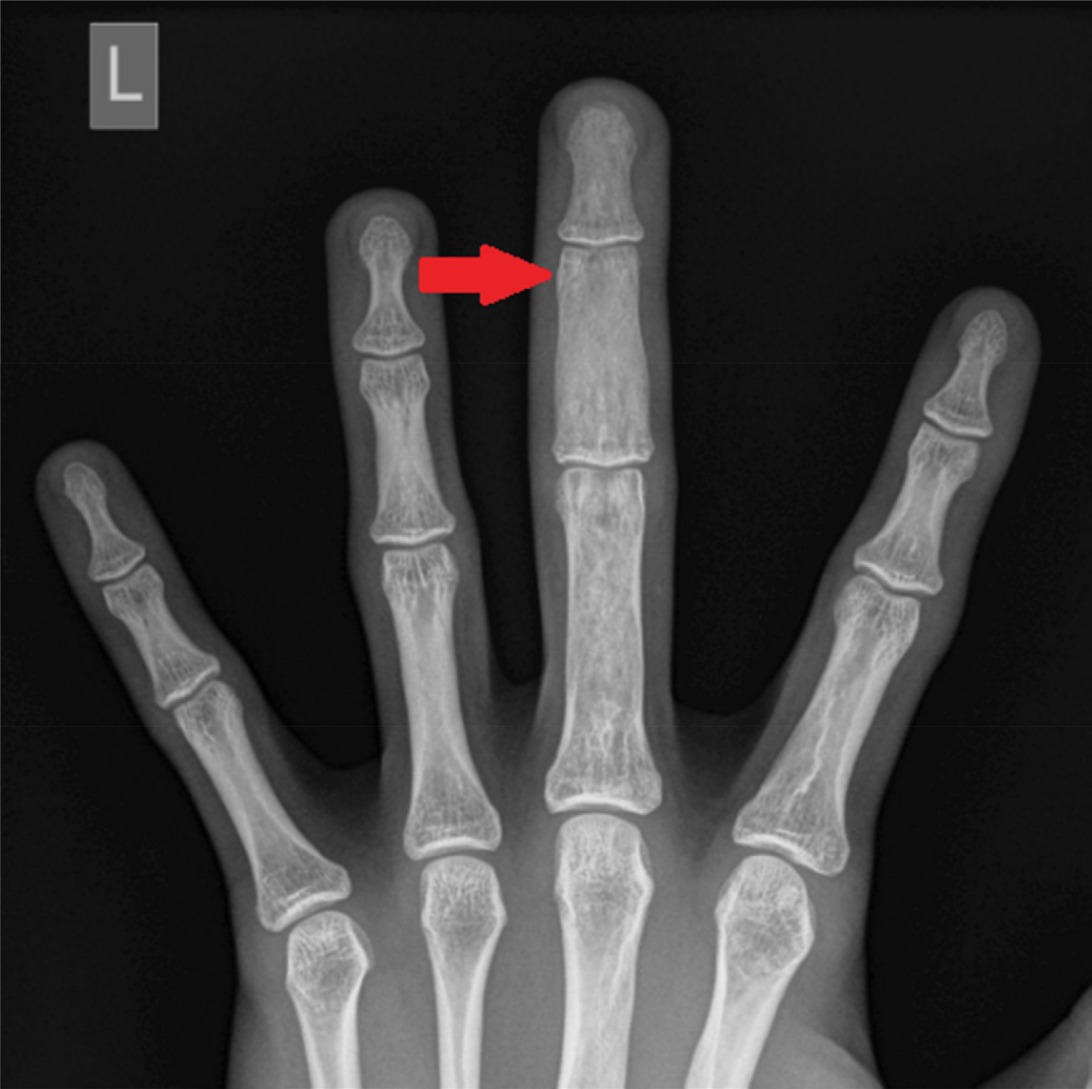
Anteroposterior X-ray of the left hand showing an expansile lesion involving all phalanges of the middle finger and a visible fracture line in the middle phalanx.
